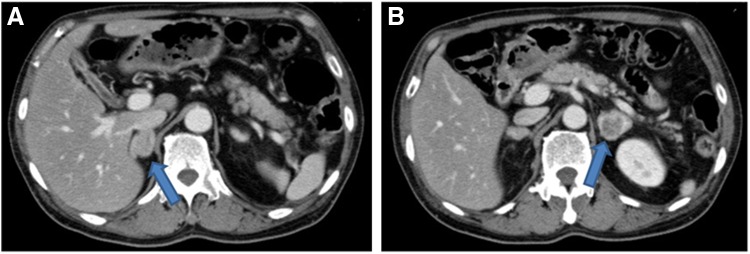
Adrenal CT scan shows right mass measuring 1.7 × 2.3 cm with mild enhancement (A) and a left mass measuring 2.8 × 3.3 cm with multiple areas of necrosis (B).
